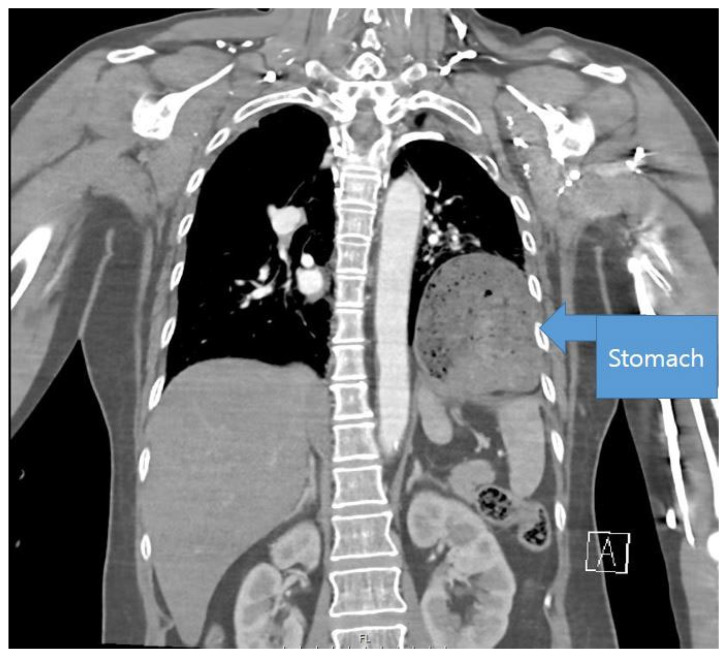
Stomach herniation. Computed tomography image showing partial herniation of the stomach into the thoracic cavity, indicating left-sided diaphragmatic injury.
